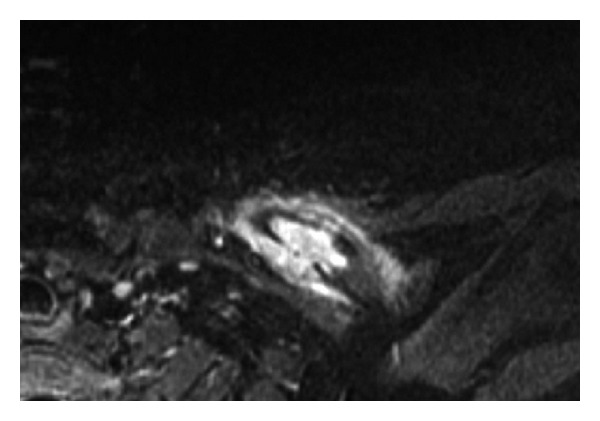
An axial STIR MR image shows a hyperintense mid-left clavicle lesion with periosteal reaction anteriorly. Moderate surrounding soft tissue hyperintense signal or edema is detected without discrete soft tissue mass or neurovascular invasion. The posterior cortical disruption is noted.
